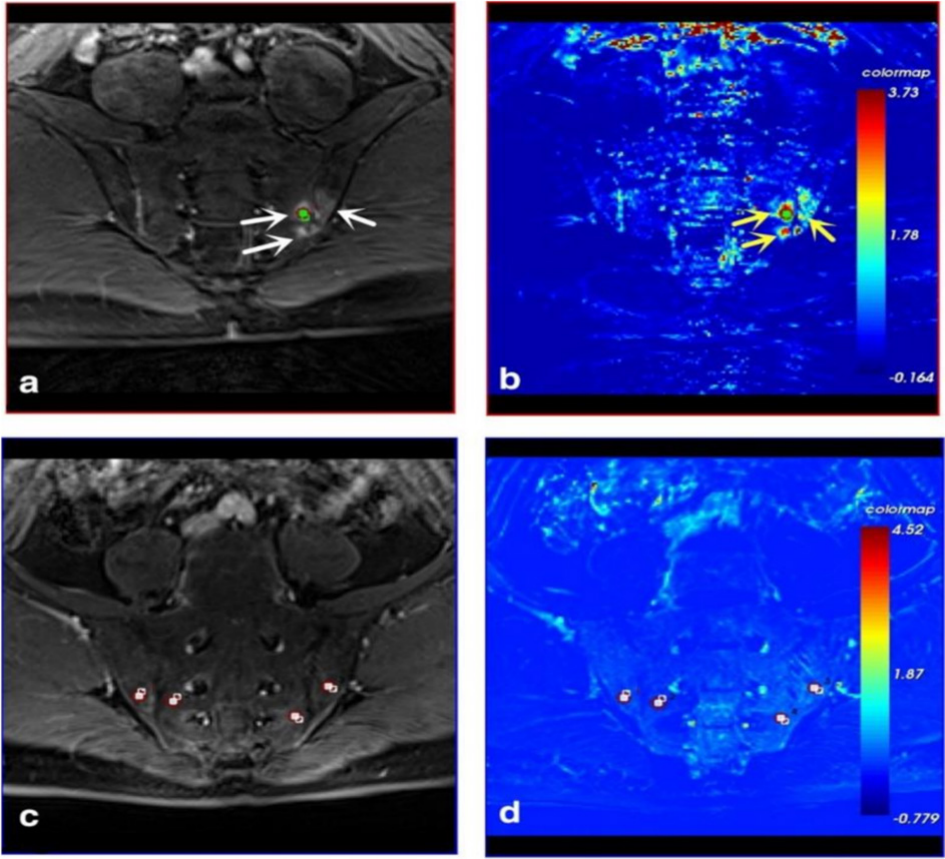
DCE-MRI of two patients with axSpA demonstrating active (a,b) and inactive (c,d) disease (figure reproduced from Zhang et al^
